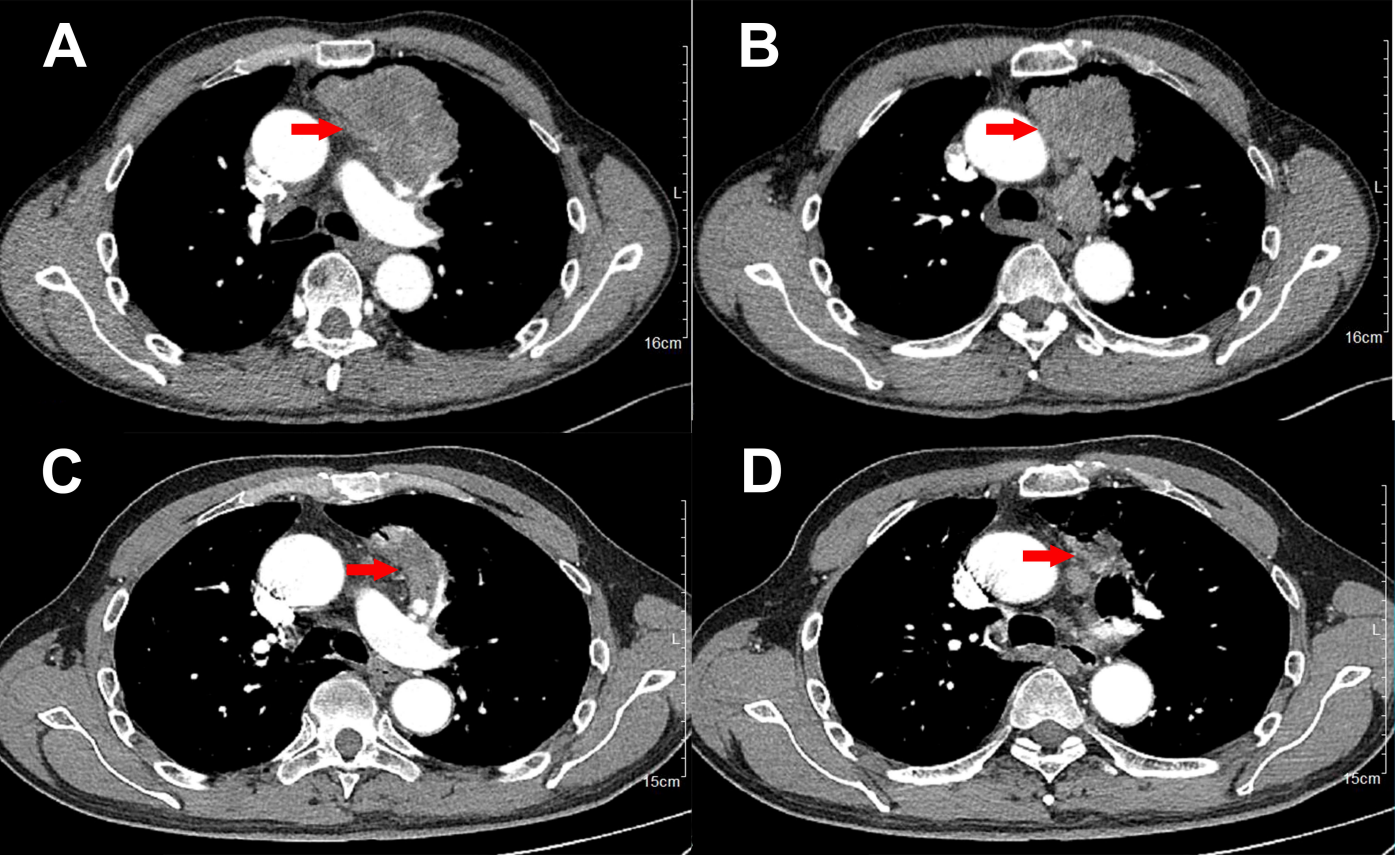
Variations of the primary lesions in lung by chest CT scans during the treatment (red arrowheads). (A, B), June 6^th^, 2022. (C, D), August 27^th^, 2022.
